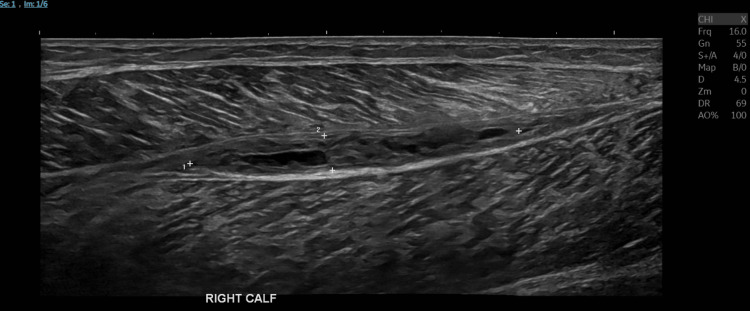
Repeat ultrasound compression venography of the right calf showing a reduction in the size of the hematoma
